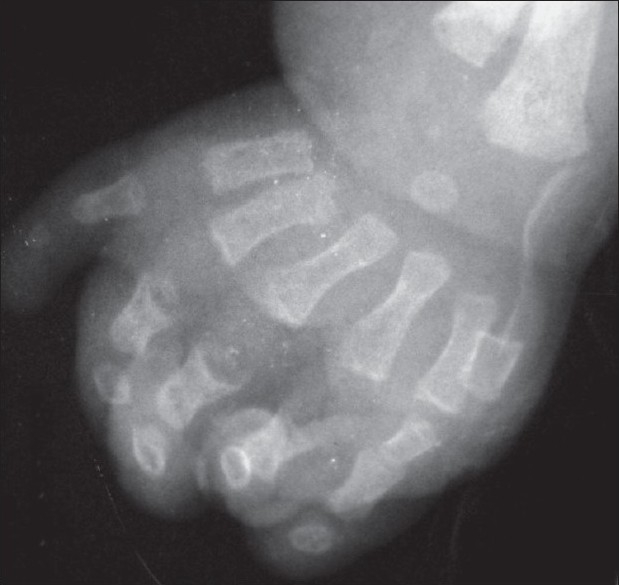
Radiograph showing shortening of distal and middle phalanges as compared to proximal ones
